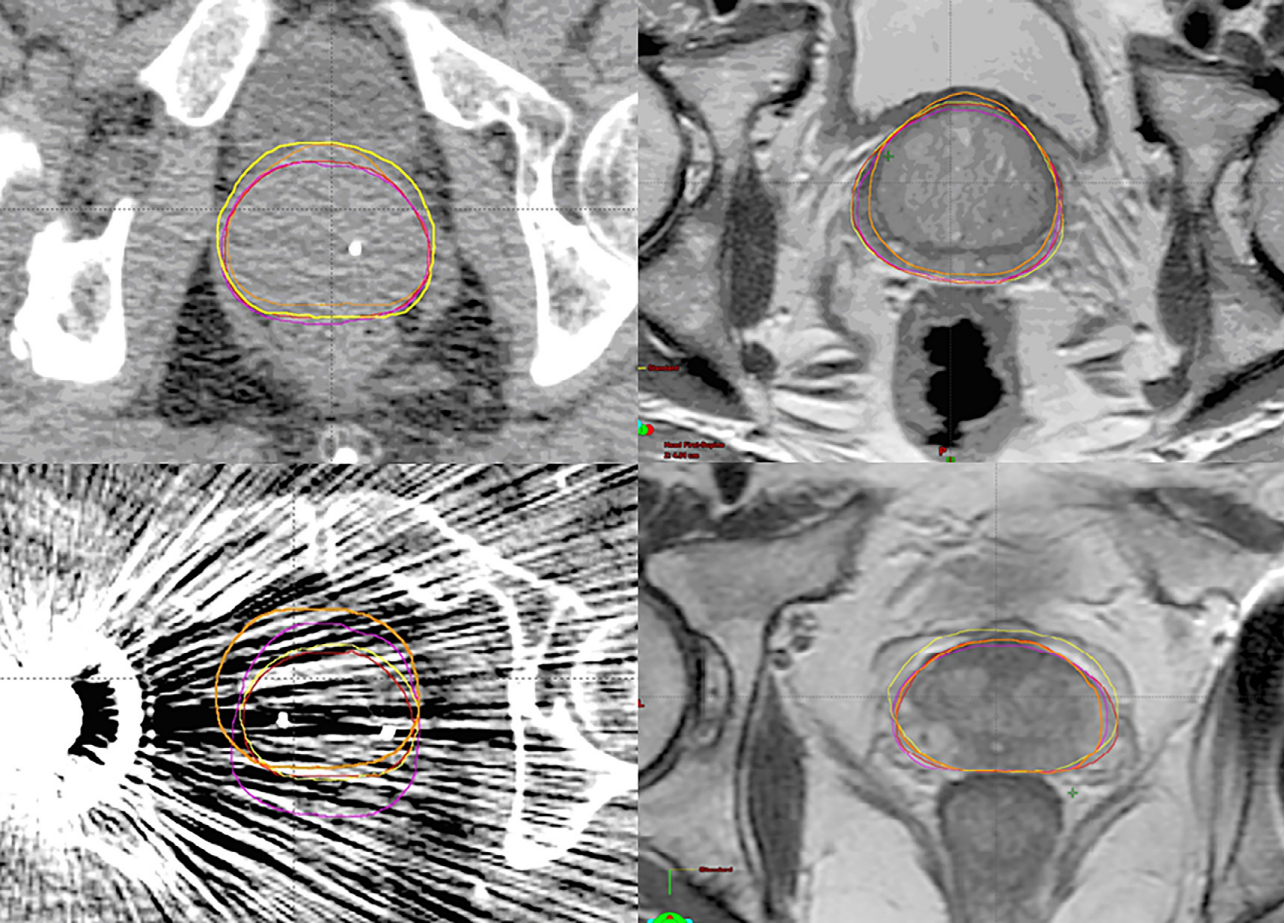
Prostate contouring at the mid‐gland level on computed tomography and magnetic resonance imaging demonstrating variability in target volume delineation between four radiation oncologists for patients 1 (above) and 3 (below).
